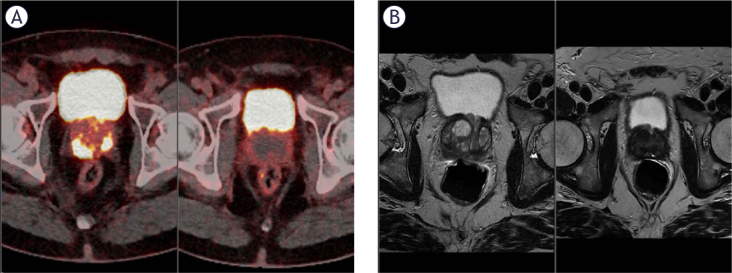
61 years old patient with biopsy-confirmed Gleason 6 multifocal prostate cancer but based on tracer uptake on prostate-specific membrane antigen (PSMA) PET and apparent diffusion coefficients (ADCs) on MRI, a higher-grade cancer. (A) Gallium-68 PSMA PET CT scan of the prostate before treatment (left) and 3 months after ECT (right). (B) MRI images of the prostate before treatment (left) and approximately 3 months after ECT (right).
